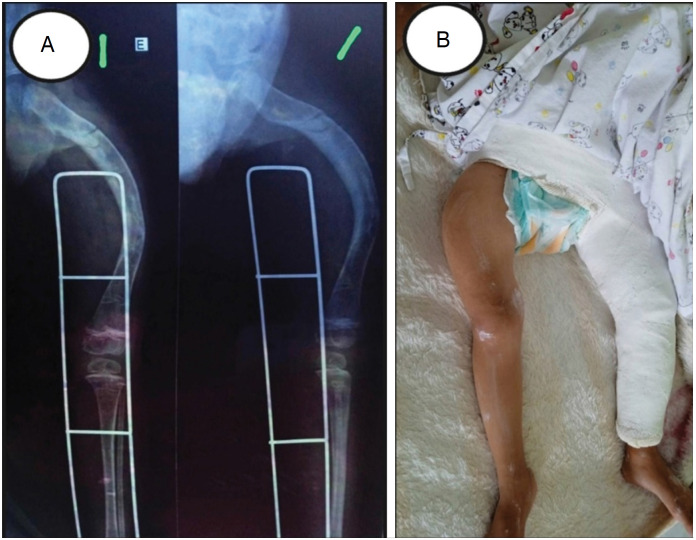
X-ray image of left femur fracture (A) and immobilized left lower limb photograph of patient with fracture (B).
